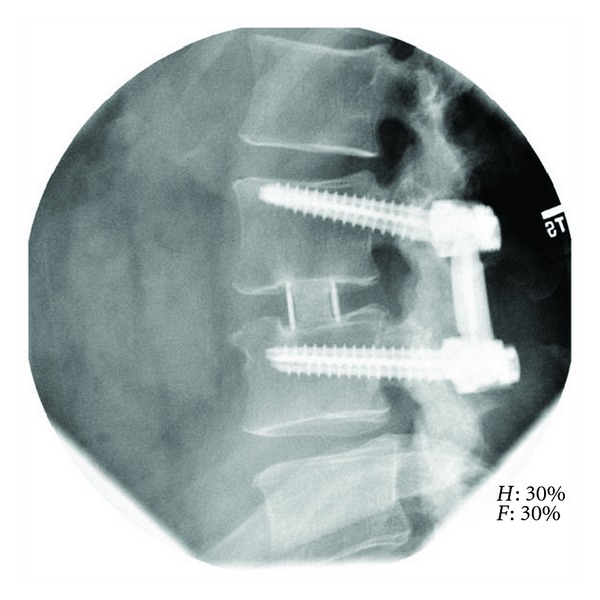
Lateral radiograph of L3-4 extreme lateral interbody fusion with Osteocel Plus at 12-month postoperatively.
